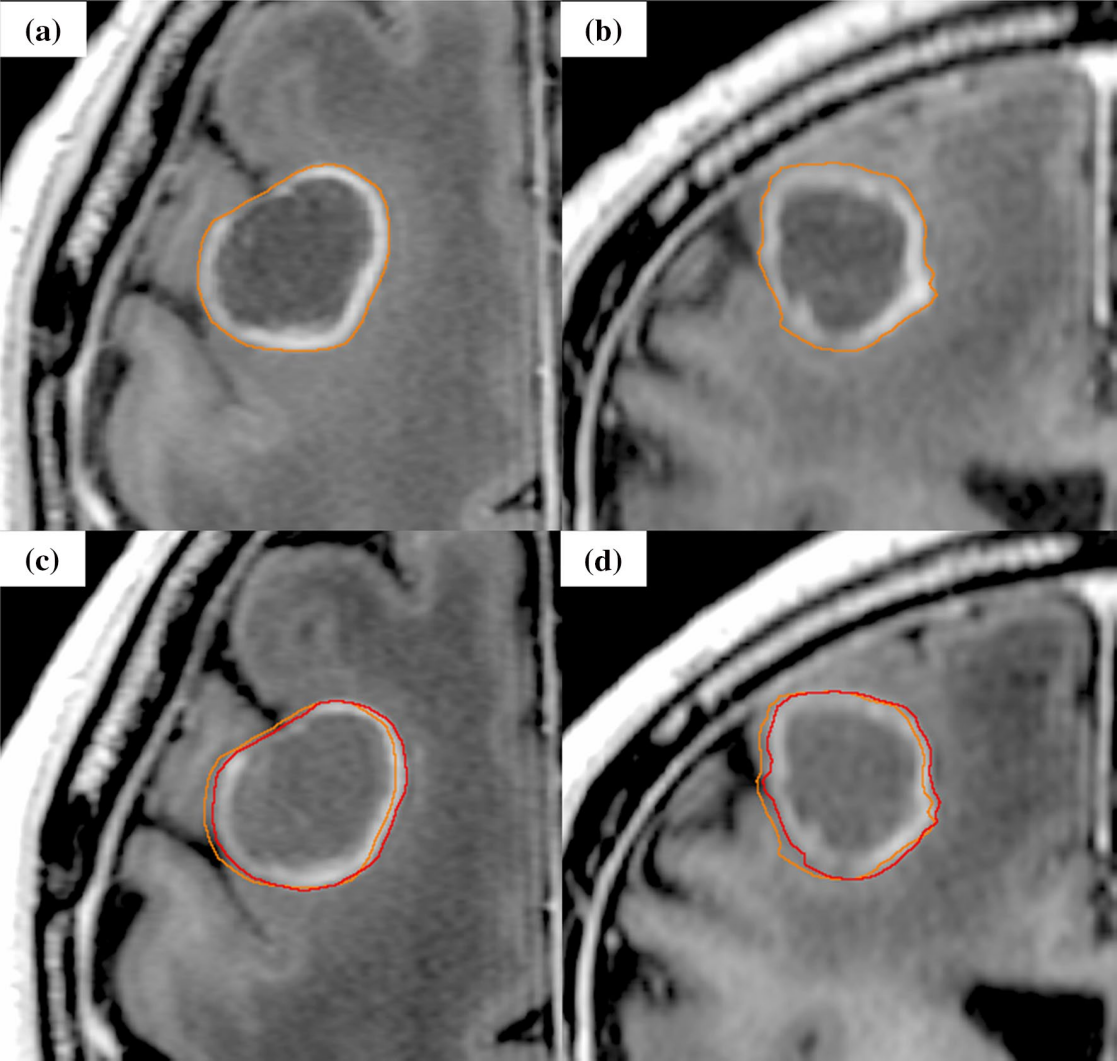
The case of displacement during stereotactic radiotherapy. The case was brain metastasis of which primary tumor was bladder urothelial carcinoma. Orange line shows the gross tumor volume (GTV) of the initial plan, and red line shows GTV at the modification. The tumor shifted to the left. a, b Axial, Coronal: T1-weighted magnetic resonance imaging (MRI) with gadolinium (Gd) of the initial plan. c, d Axial, Coronal: T1-weighted MRI with Gd before third treatment for the modification (the third day of SRT start)
